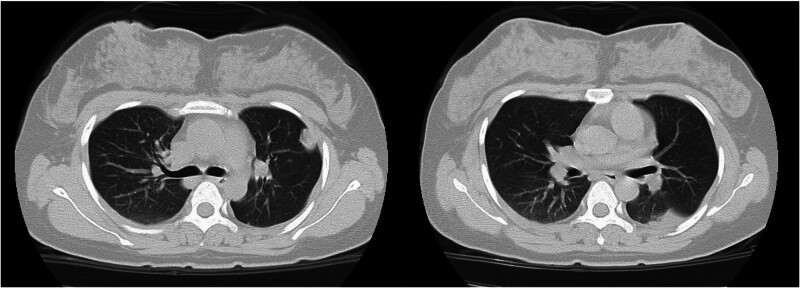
Ten days later, 16-slice computed tomography reexamination images of the chest revealed that the shadow in the patient’s lungs decreased and became lighter in the left lung after treatment with a combination of antibiotics and methylprednisolone.
